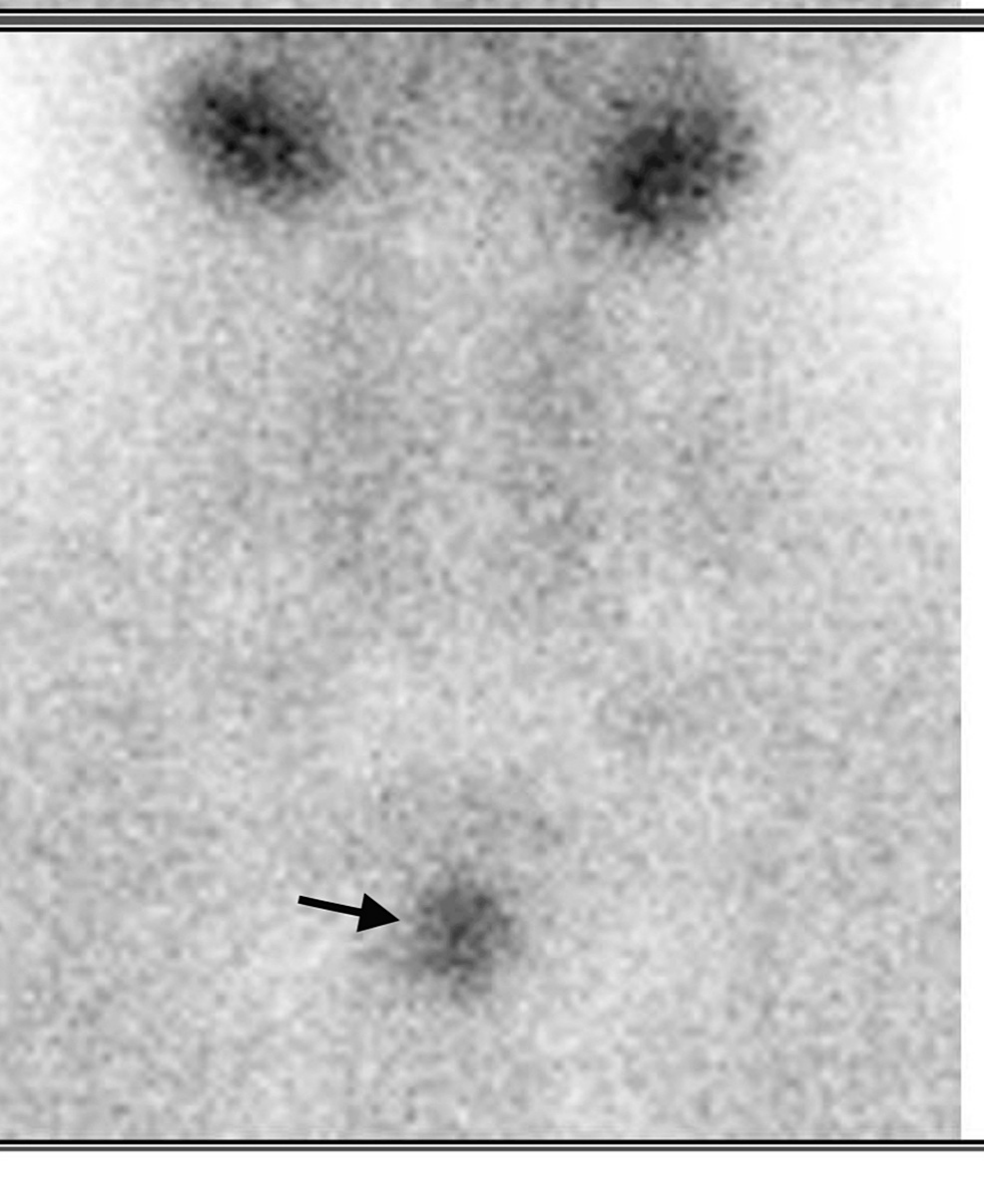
Parathyroid scintigraphy with 622 MBq of Tc-99m sestamibi injected intravenously. Solitary parathyroid adenoma posterior to manubrium sterni (ectopic position).
